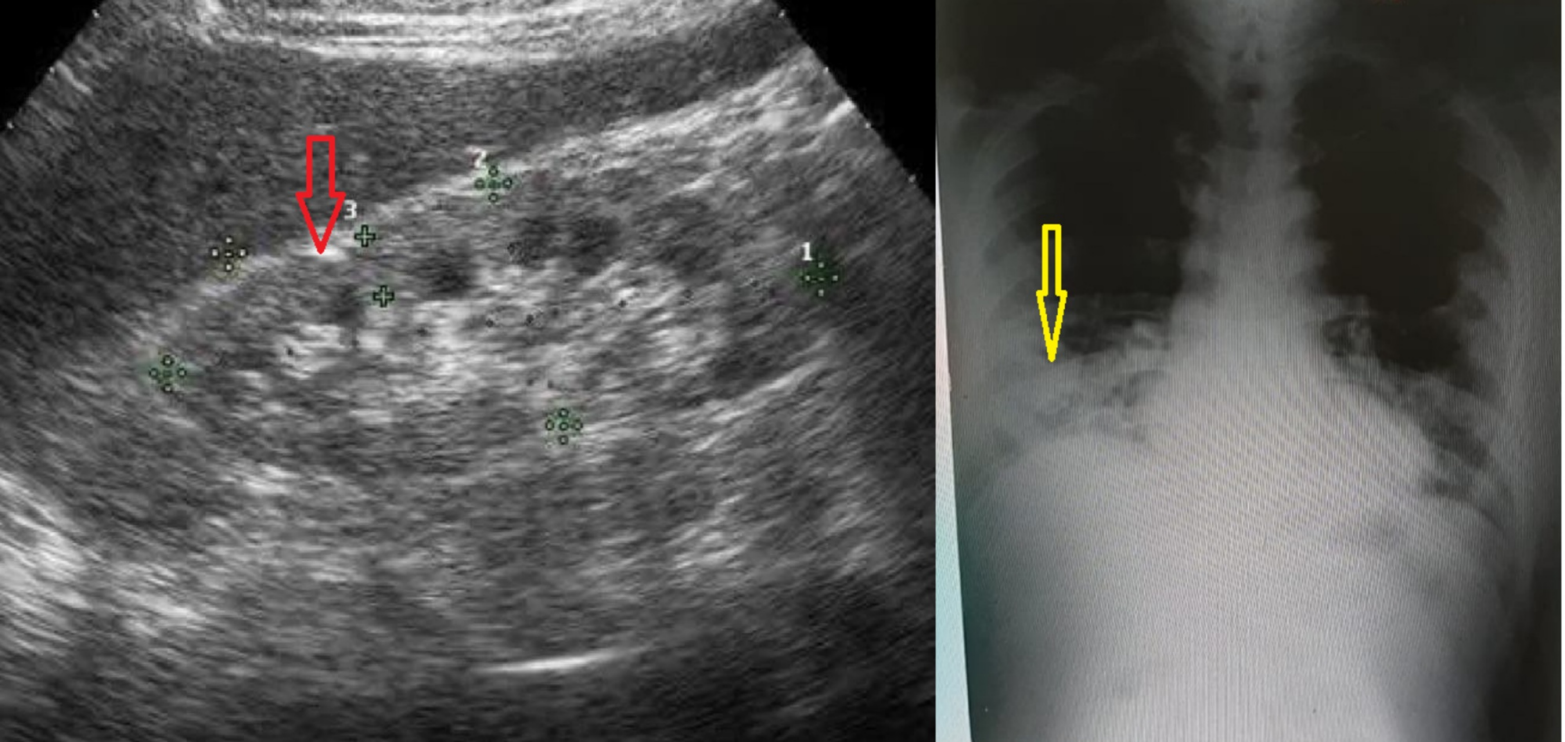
Chest x-ray (CXR) on the day of admission showed a right basal pneumonic patch (yellow arrow). Kidney ultrasound showed normal-sized kidneys with grade one echogenicity and no hydronephrosis (red arrow)
